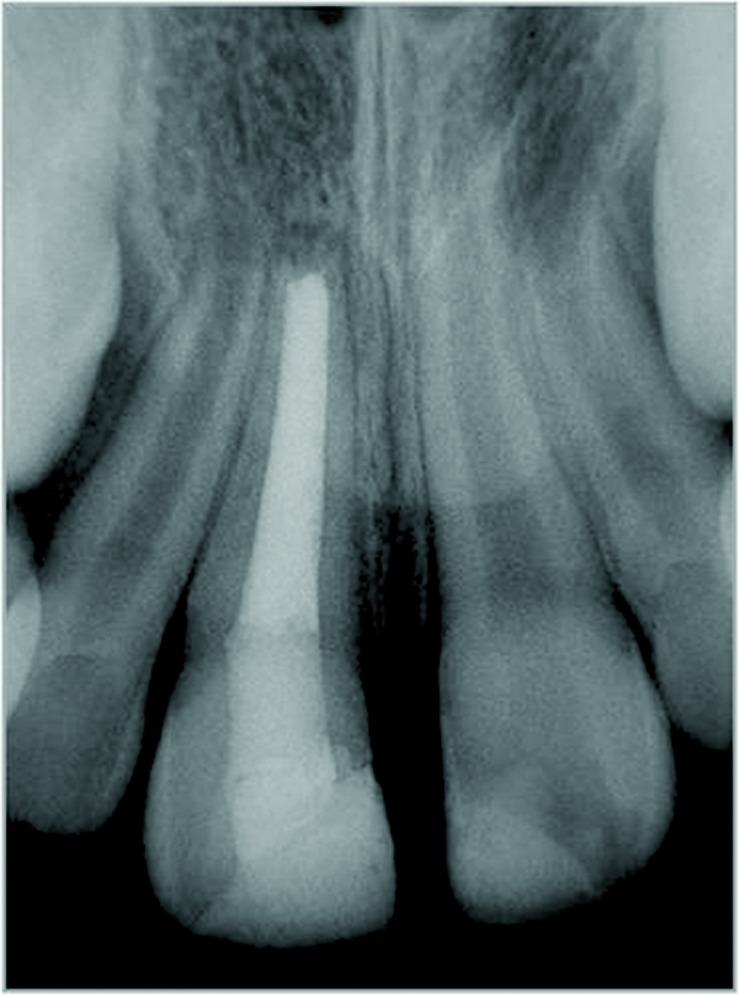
Six-month follow-up radiograph reveals a mineralized bridge in the apical region
